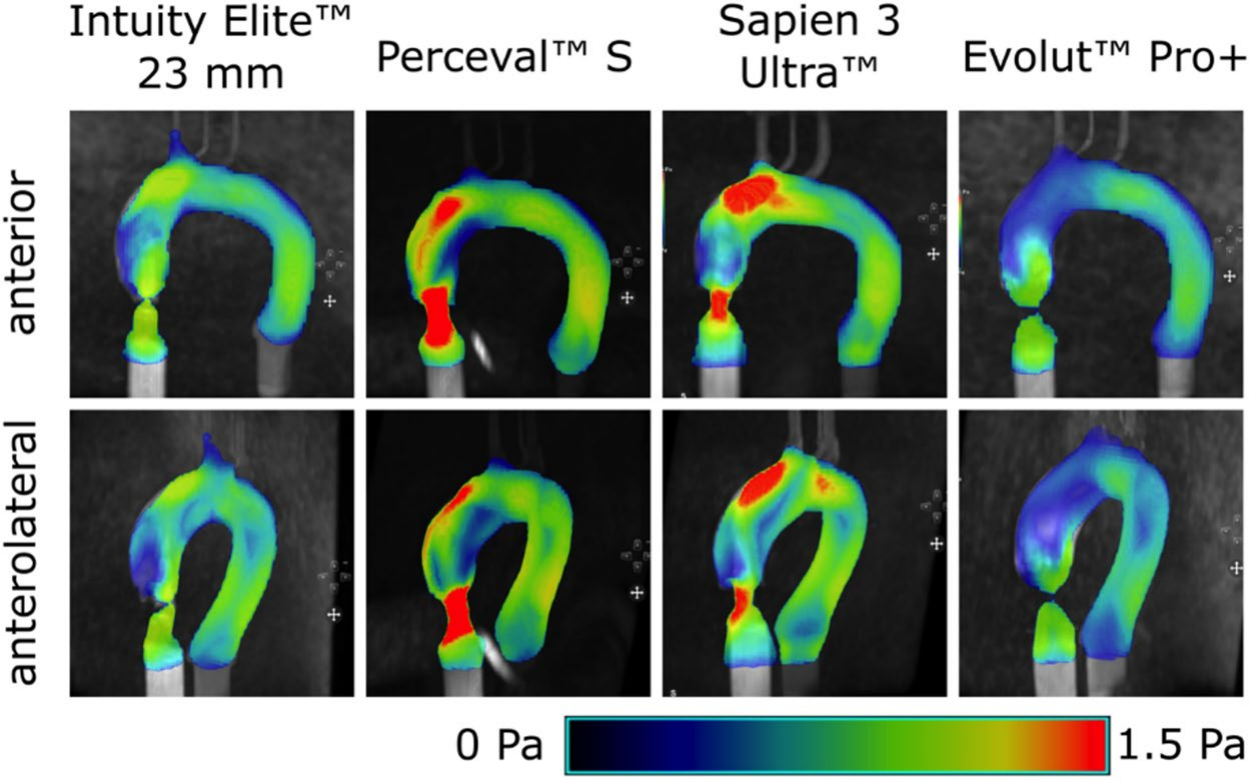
Qualitative wall shear stress distribution during peak systole of the cardiac cycle visualized in anterior and anterolateral view in 4D flow Magnetic Resonance Imaging.
